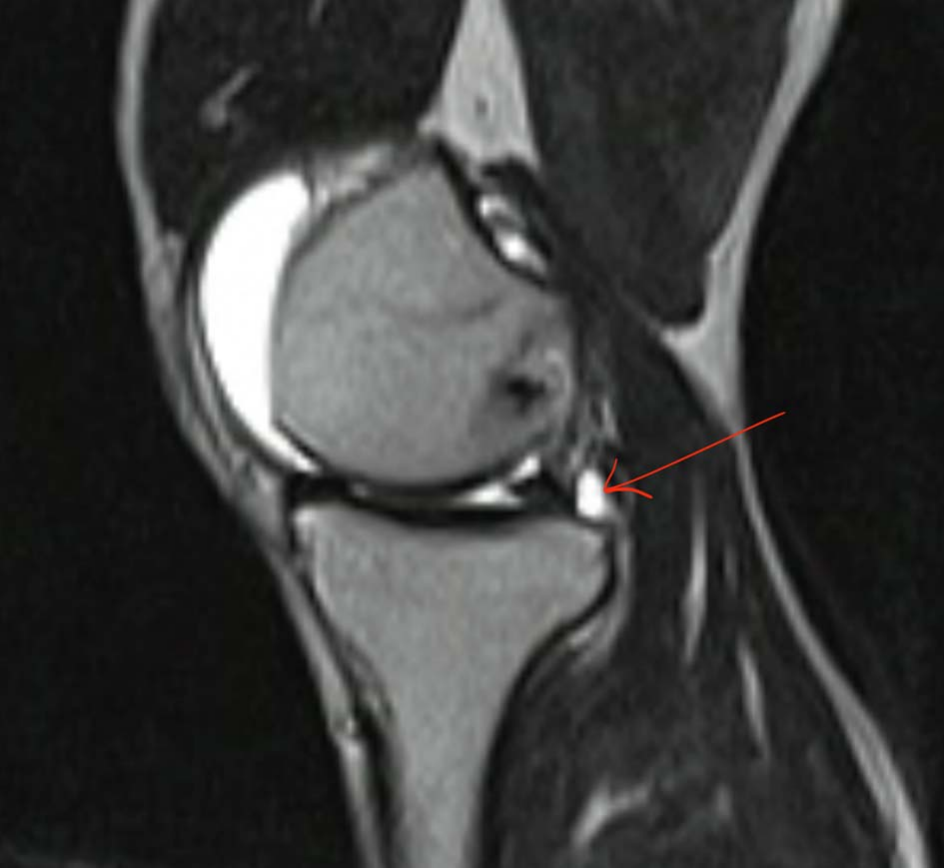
The MRI of the medial meniscus (the T2W MRI showed that the red arrow indicated a tear in the posterior horn of the medial meniscus).
